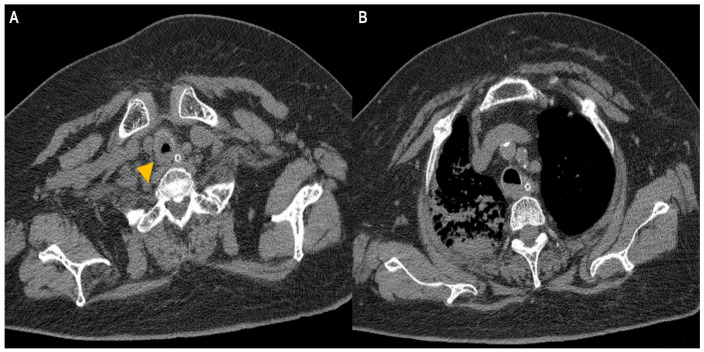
Chest computed tomography images. (A, arrowhead) Aggravated diffuse wall thickening with luminal narrowing at the proximal trachea. (B) Peribronchial consolidation and secretion stasis in the right upper lobe.
